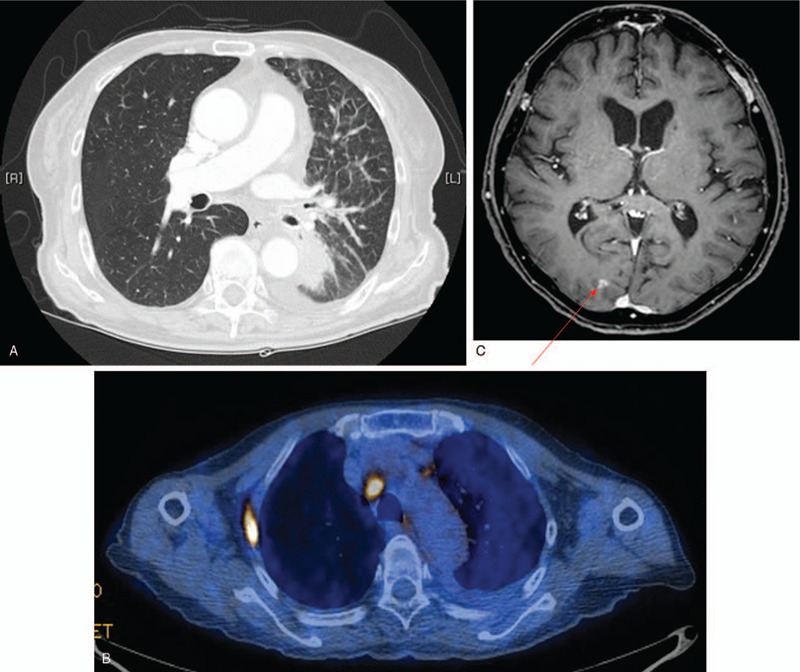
(A) A chest computed tomography scan on January 2, 2014, revealed a mass with a long axis of 3.9 cm in the left lower lobe with lymphangitic metastasis and pleural effusion. (B) A positron emission tomography/CT scan on January 4, 2014, showed bone metastasis in the right third and tenth ribs. (C) A brain magnetic resonance image on January 2, 2014, showing the cortical metastasis. The red arrow indicates a metastatic lesion with high signal intensity.
